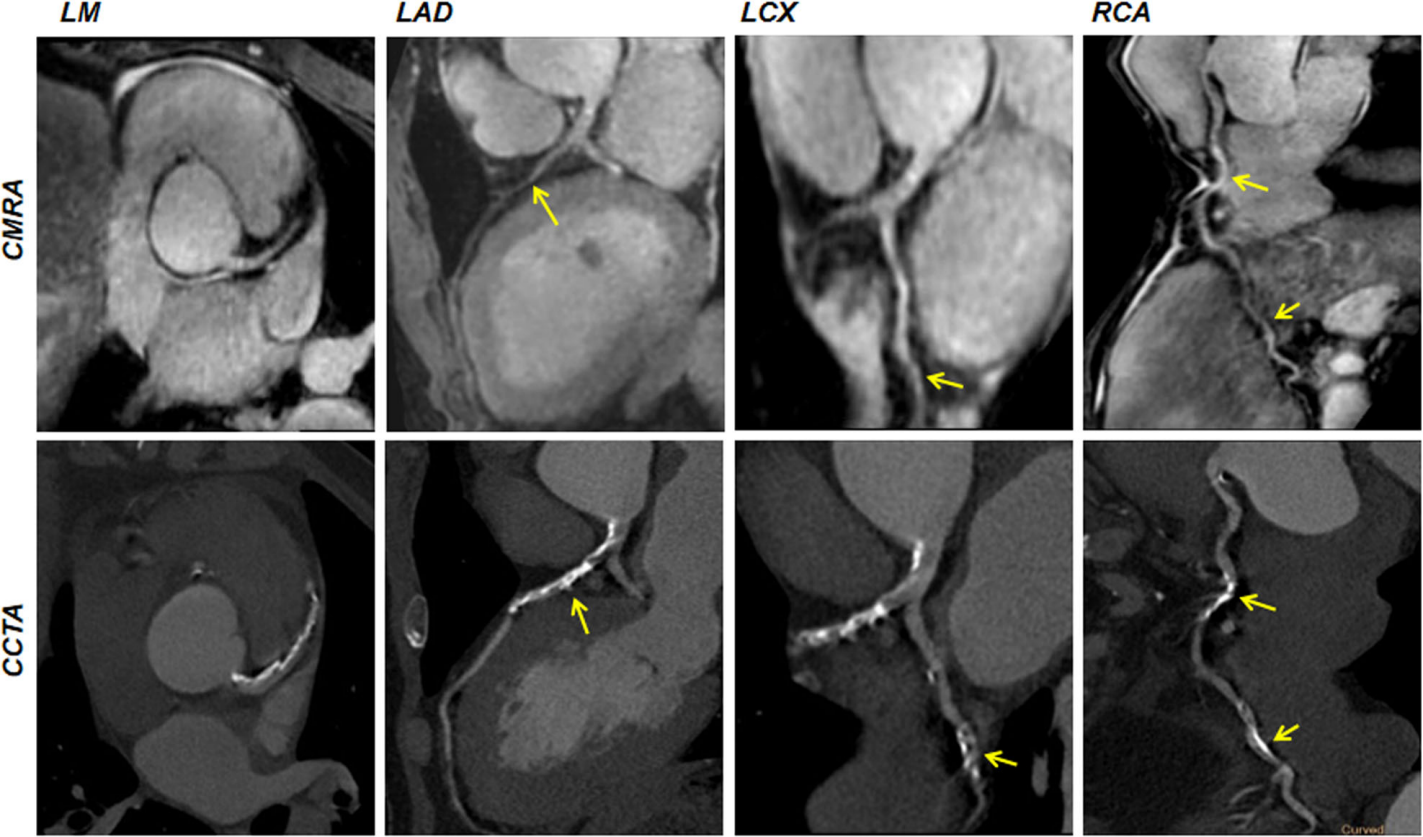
Curved planar reformation coronary MRA and CCTA images from a 63-year-old male patient with diffuse calcification and significant stenosis (≥ 50% diameter stenosis) (yellow arrows). Coronary MRA images show stenosis matching the lesion detected by CCTA. CCTA, coronary computed tomography angiography; CMRA, coronary magnetic resonance angiography; LAD, left anterior descending artery; LCX, left circumflex; LM, left main artery; RCA, right coronary artery
